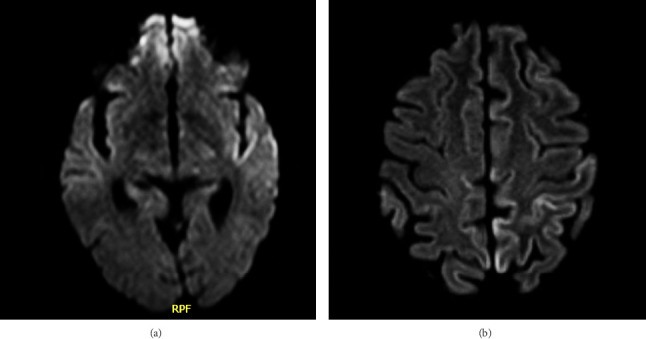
(a) MRI from initial presentation showing mild restricted diffusion and T2/FLAIR hyperintense signal involving the left insula and anterior temporal lobe cortex. (b) Repeat MRI 4 weeks later showing abnormal diffusion and T2 FLAIR signal in the cerebral cortex bilaterally compatible with cortical ribboning.
